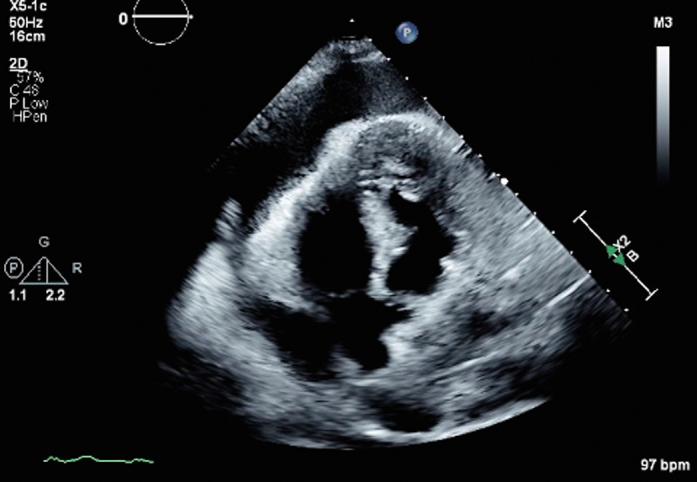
Apical 4-Chamber View Revealing Large Pericardial Effusion and Systolic Right Atrial Collapse
